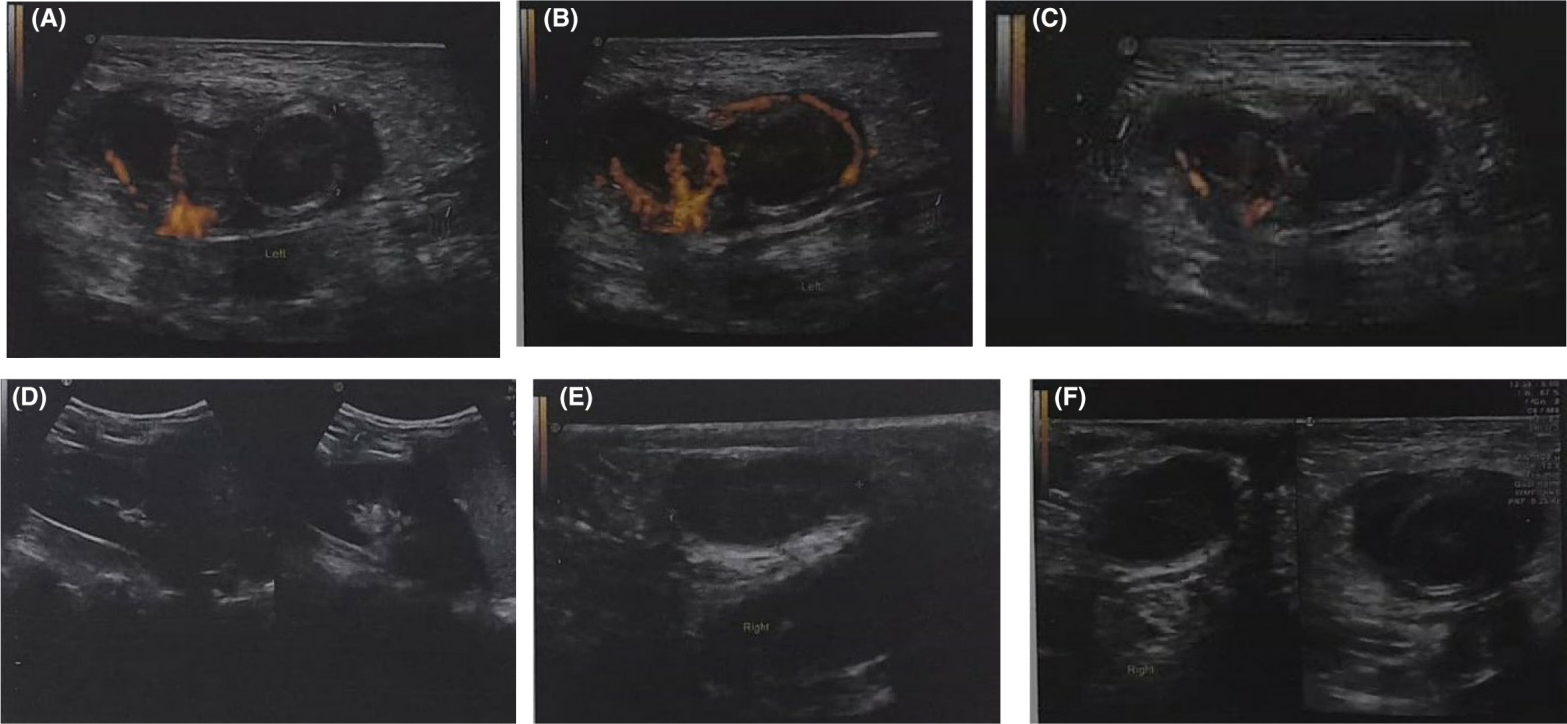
(A–C) The Doppler color scan demonstrated that the left testis in the inguinal area had a hyperechoic pattern, no blood flow, and excess fluid around it, and the left epididymis had a high blood flow and a hypoechoic pattern. (D, E) These figures demonstrate the right testis and epididymis by ultrasonography. (F) This figure demonstrates the difference between right and left testis.
